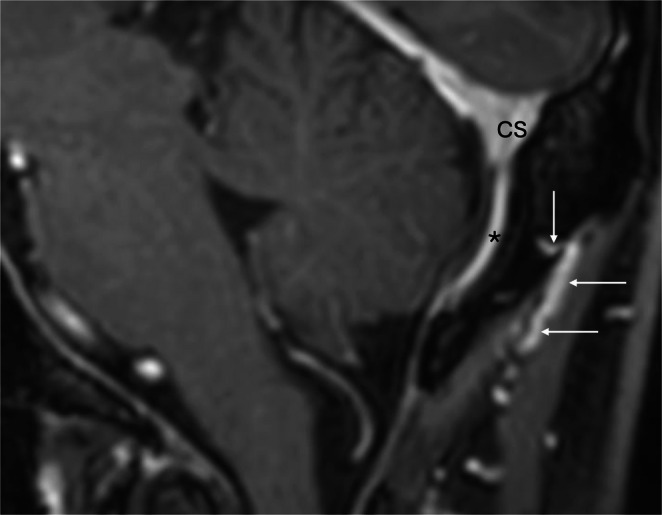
Sagittal contrasted MRI noting an occipital vein (two horizontal arrows), emissary vein (vertical arrow) entering the diploic space, and occipital sinus (*) and confluence of sinuses (CS) (torcular Herophili)
